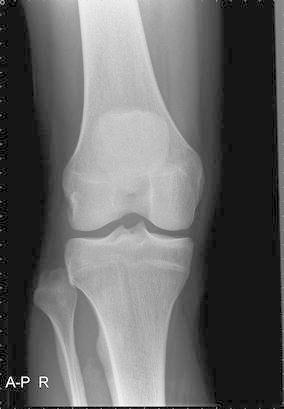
AP X-ray of the right knee. Based on this X-ray, the diagnosis of luxation of the fibula was not made
